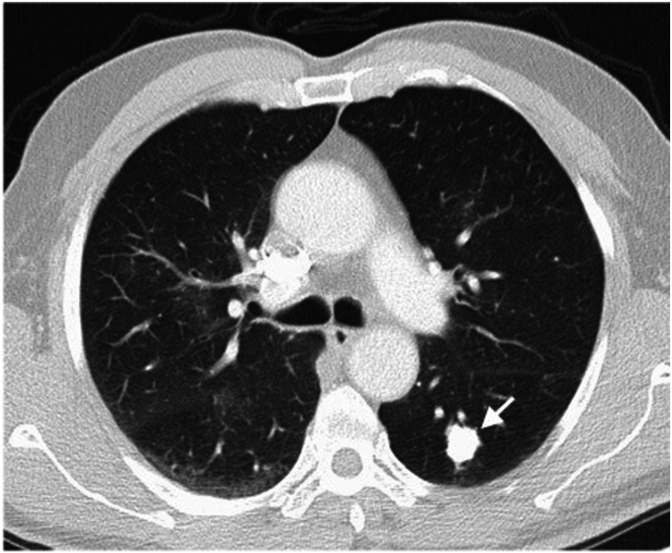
CT Chest revealing calcified left lower lobe nodule (arrow).
